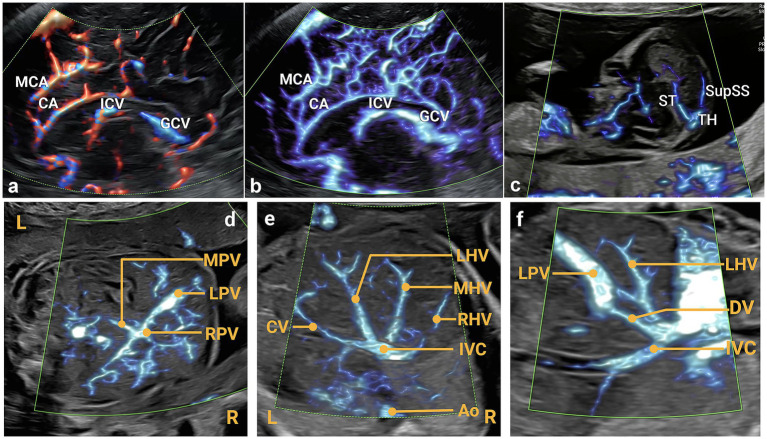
(a–c) Compare brain vasculature imaged with HD-Doppler vs. Slow*flow*HD. In this normal fetus imaged at 34 weeks GA, note the marked difference in the quantity and scale of the vessels imaged and their clarity, comparing HD-Doppler (a) and Slow*flow*HD (b). In early gestation, vasculature not usually amenable to imaging is seen in detail. In this 13-weeks fetus, the straight sinus (ST), superior sagittal sinus (SupSS), and even the Torcular Herophili (TH) are imaged (c). (MCA, mid-cerebral artery; CA, callosal artery; ICV, internal cerebral vein; GCV, great cerebral vein). (d–f) The three planes of the precordial venous system imaged in Slow*flow*HD. (a) The lateral transverse plane through the fetal abdomen from left to right. (LPV; left portal vein; MPV, main portal vein; RPV, right portal vein. L and R indicate left and right). (b) Anterior–posterior transverse plane through the upper fetal abdomen showing the normal hepatic veins, and to the left of the LHV, the caudate lobe vein. (Ao, aorta; CV, caudate vein; LHV, left hepatic vein; MHV, middle hepatic vein; RHV, right hepatic vein. L and R indicate left and right). (c) Typical longitudinal plane showing the left portal vein (LPV); left hepatic vein (LHV); ductus venosus (DV); and inferior vena cava (IVC).
